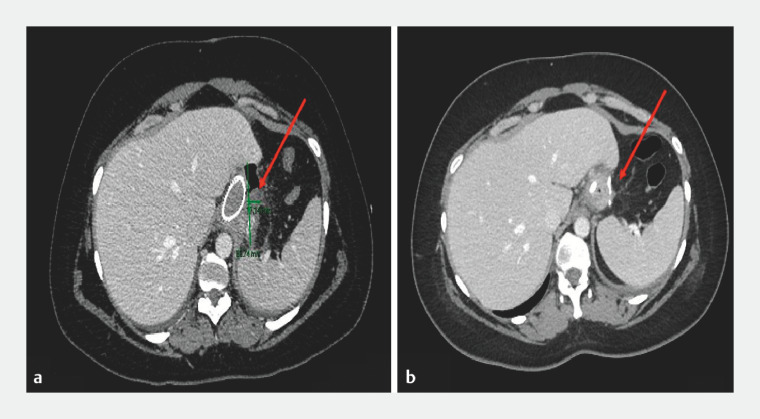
Computed tomography showing (arrowed):
